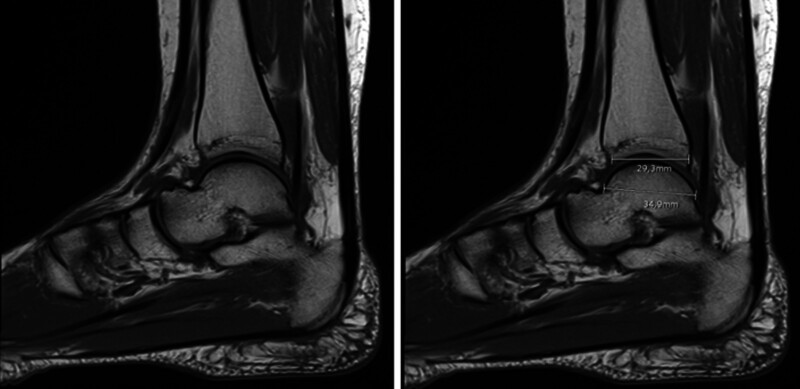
The span of the distal tibial articular surface (TAS) in the sagittal plane and the extent of the trochlea tali arc (TAL) were quantified from sagittal T1-weighted images. TAS was assessed by measuring the most anterior to the most posterior point on the distal tibial articular surface, whereas TAL was determined by the distance between the farthest anterior and posterior points of the trochlea tali. The TAS/TAL ratio was generated to standardize these dimensions.
